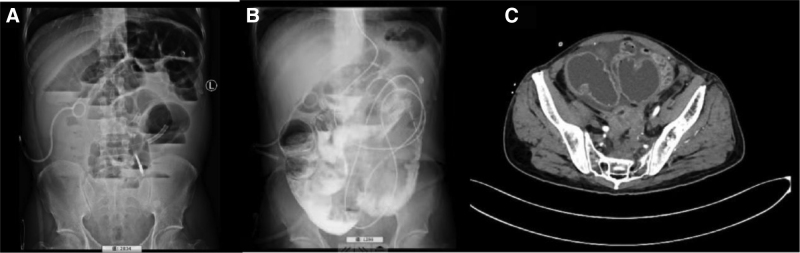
Multisegmental ileus and endoscopic enterocolostomy are possible. A, Small bowel decompression catheter placement to relieve obstruction symptoms. B, Gastrointestinal iodine contrast showing ileal and ascending colon multisegmental obstruction. C, CT showing pelvic ileal dilatation; endoscopic enterostomy is possible. CT, computed tomography.
